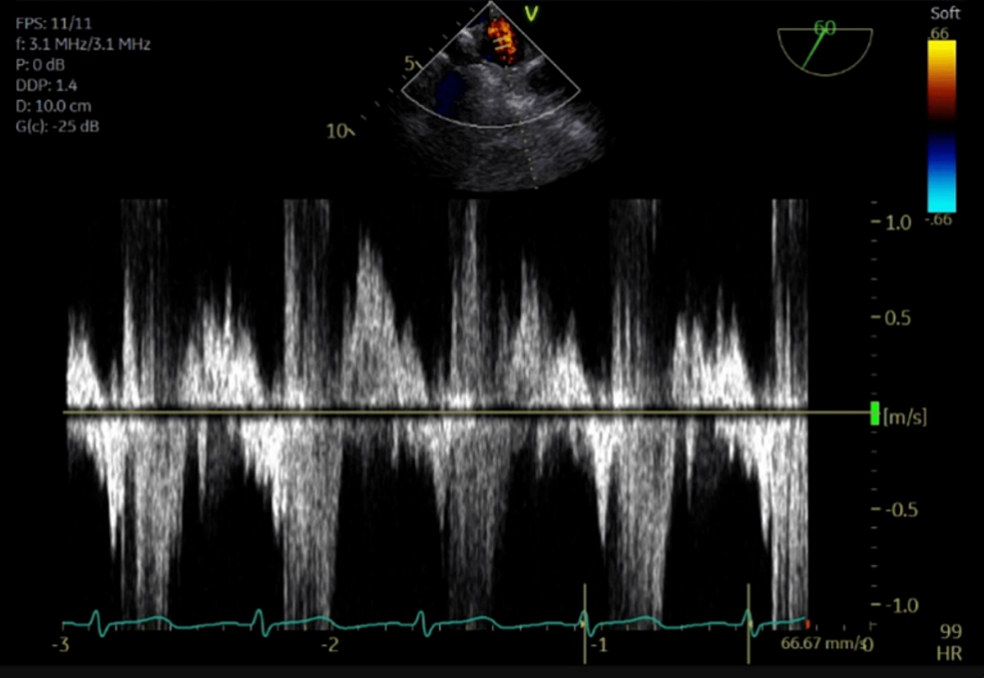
Pulsed-wave Doppler of the right superior pulmonary vein showing systolic flow reversal.
